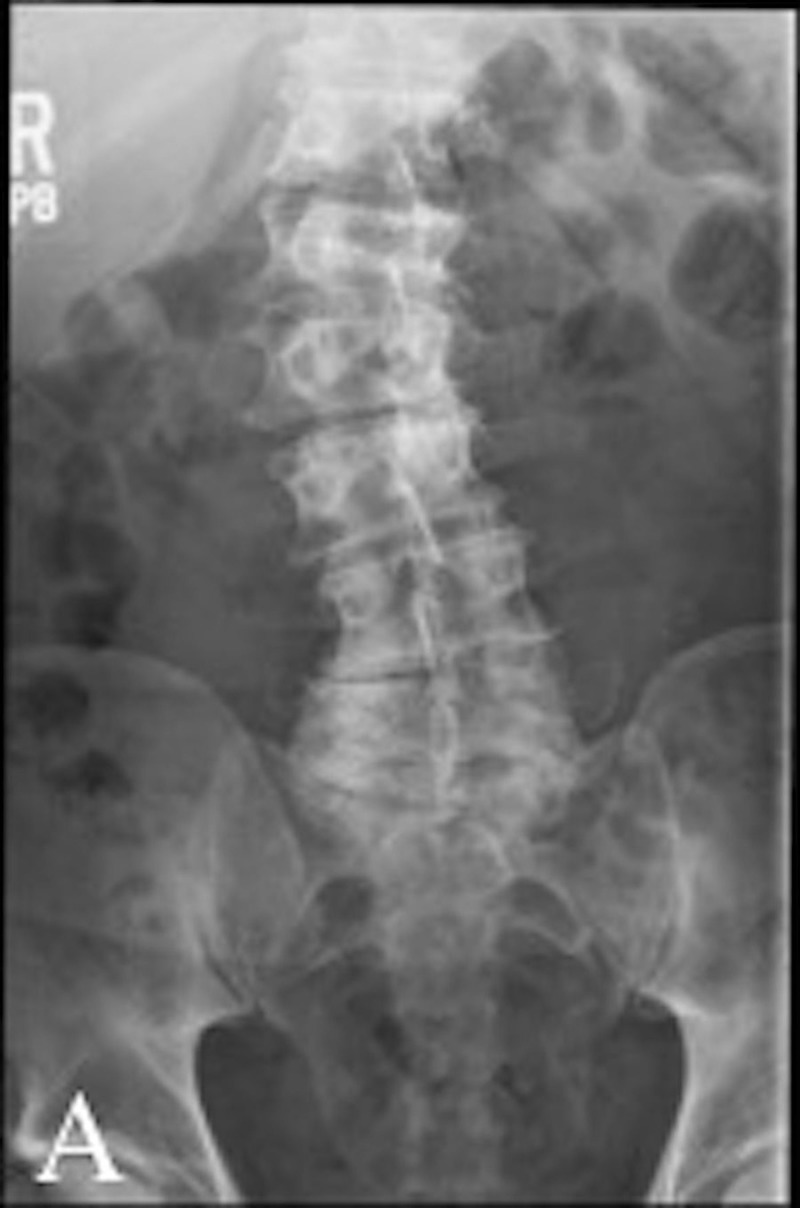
Pre-operative anterior/posterior X-rays. Pre-operative anterior/posterior X-rays demonstrating multi-level disc degeneration and neuroforaminal stenosis most severe in the lower lumbar spine.
